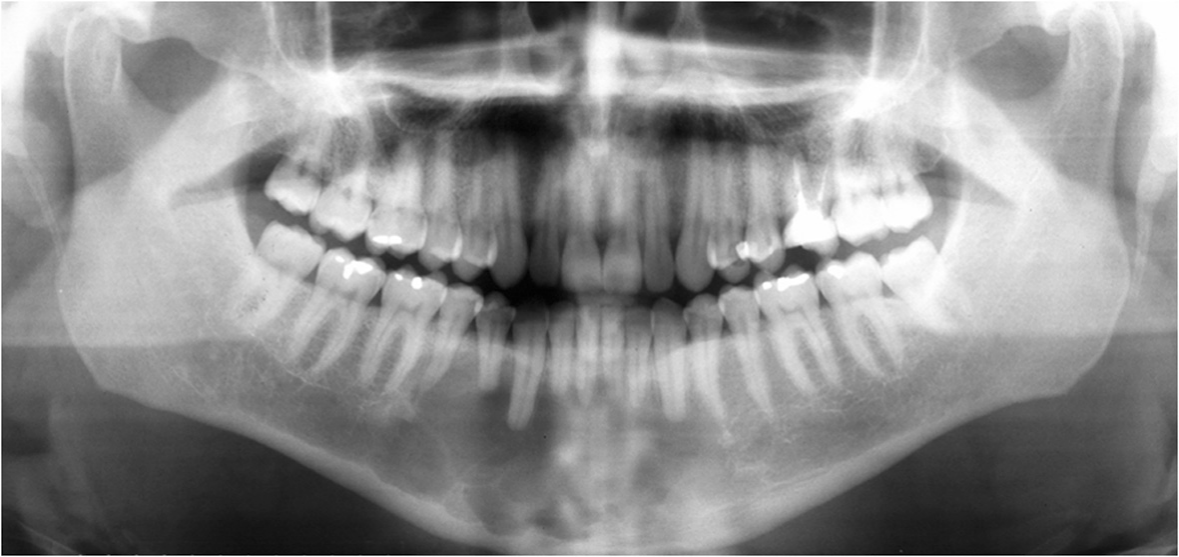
A Panoramic radiography. A multilocular radiolucent area crossing the midline and causing resorption in certain teeth.
